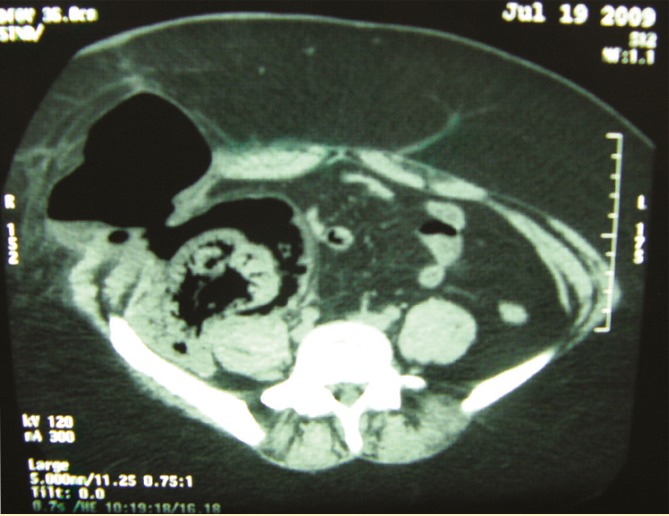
Non-contrast CT scan of the abdomen showing extensive extraperitoneal perinephric gas extending to paranephric space accompanied by renal parenchymal destruction
